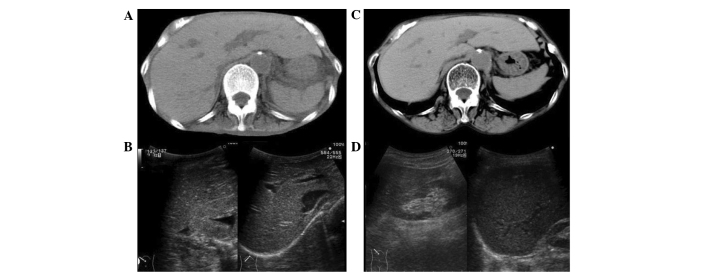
Imaging results of the patient’s liver. (A) Computed tomography and (B) ultrasound findings on admission; and (C) computed tomography and (D) ultrasound findings after 1 year.
